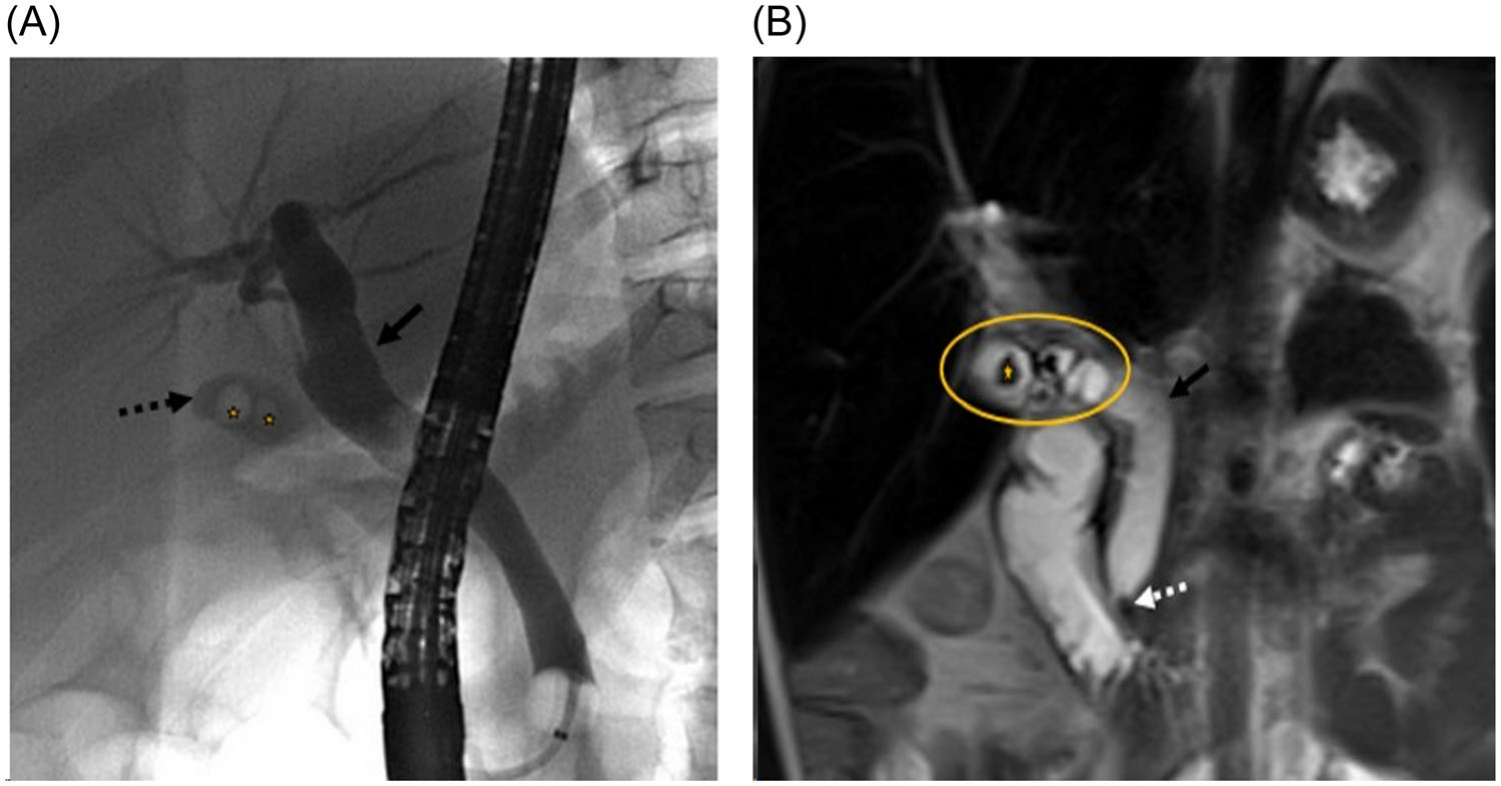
(A) Endoscopic retrograde cholangiopancreatography (ERCP) with contrast opacified biliary system. Imaging done 2 years after 1st cholecystectomy (before repeat cholecystectomy). Two lucent filling defects (starred) in the gallbladder remnant (dashed arrow). (B) Magnetic resonance cholangiopancreatography (MCRP) imaging done 2 years after 1st cholecystectomy (before repeat cholecystectomy). Cor T2‐weighted MR image shows dilated common bile duct (arrow) with hypointense calculus at the ampulla (dashed arrow). Ovoid fluid filled gallbladder remnant (circled) contains numerous additional hypointense calculi (starred).
